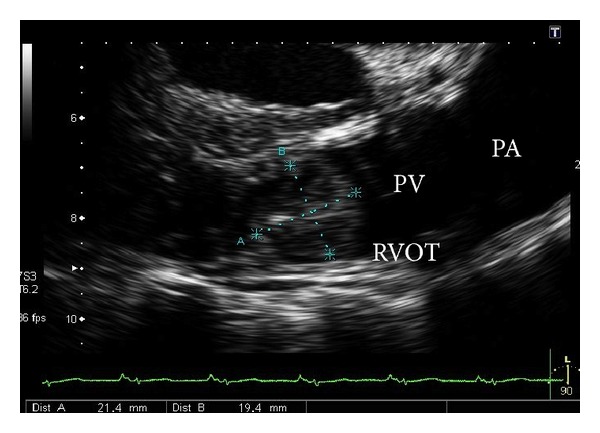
Transesophageal echocardiography shows the tumor in the right ventricular outflow tract. PA: pulmonary artery, RVOT: right ventricular outflow tract, and PV: pulmonary valve.
